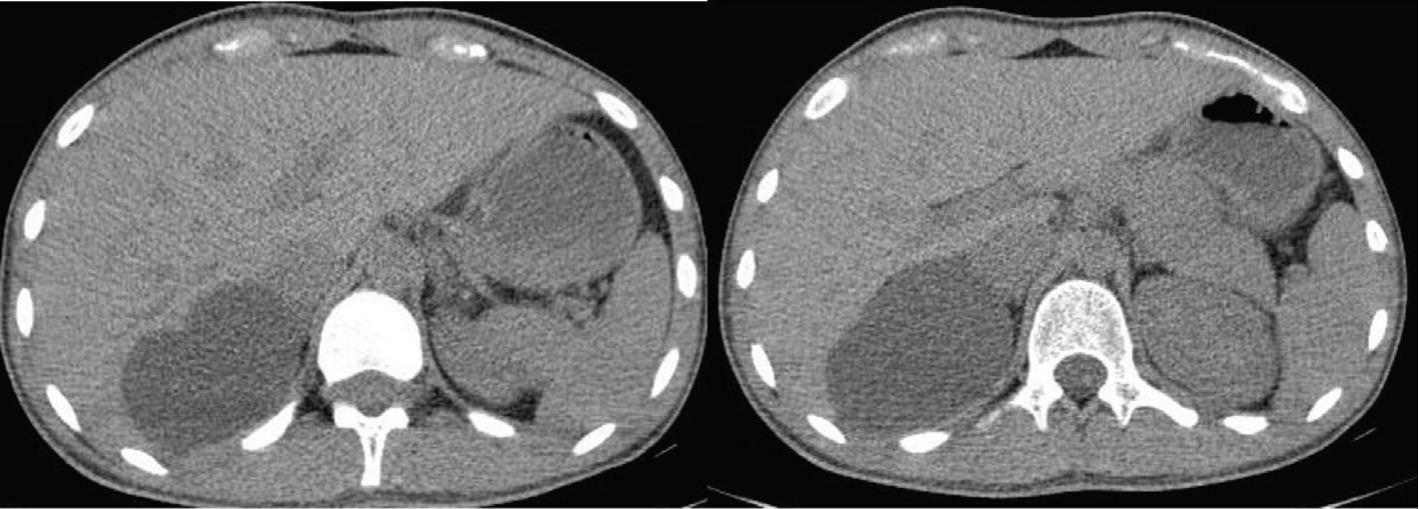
Sagittal CT image showing an 80 × 50 mm cystic lesion located primarily between the liver and right kidney. The lesion causes compression of the right liver lobe.
